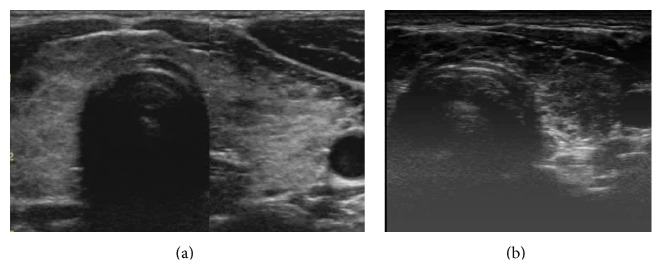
(a) Hashimoto's thyroiditis with mild sonographic hypoechogenicity. (b) Hashimoto's thyroiditis with severe sonographic hypoechogenicity.
